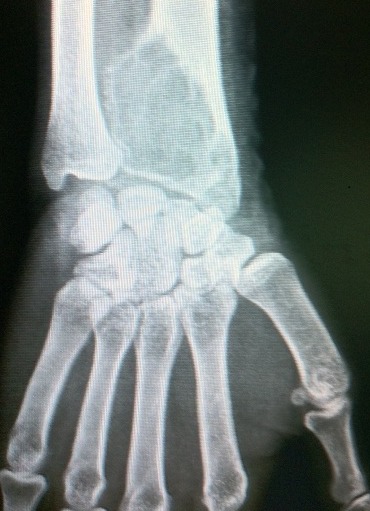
Radiographie du poignet de face montrant une volumineuse lacune multi-kystique de l'extrémité inférieure du radius, épiphyso-métaphysaire, bien limitée, « soufflant l'os » sans rupture de la corticale osseuse, envahissant la membrane interosseuse
